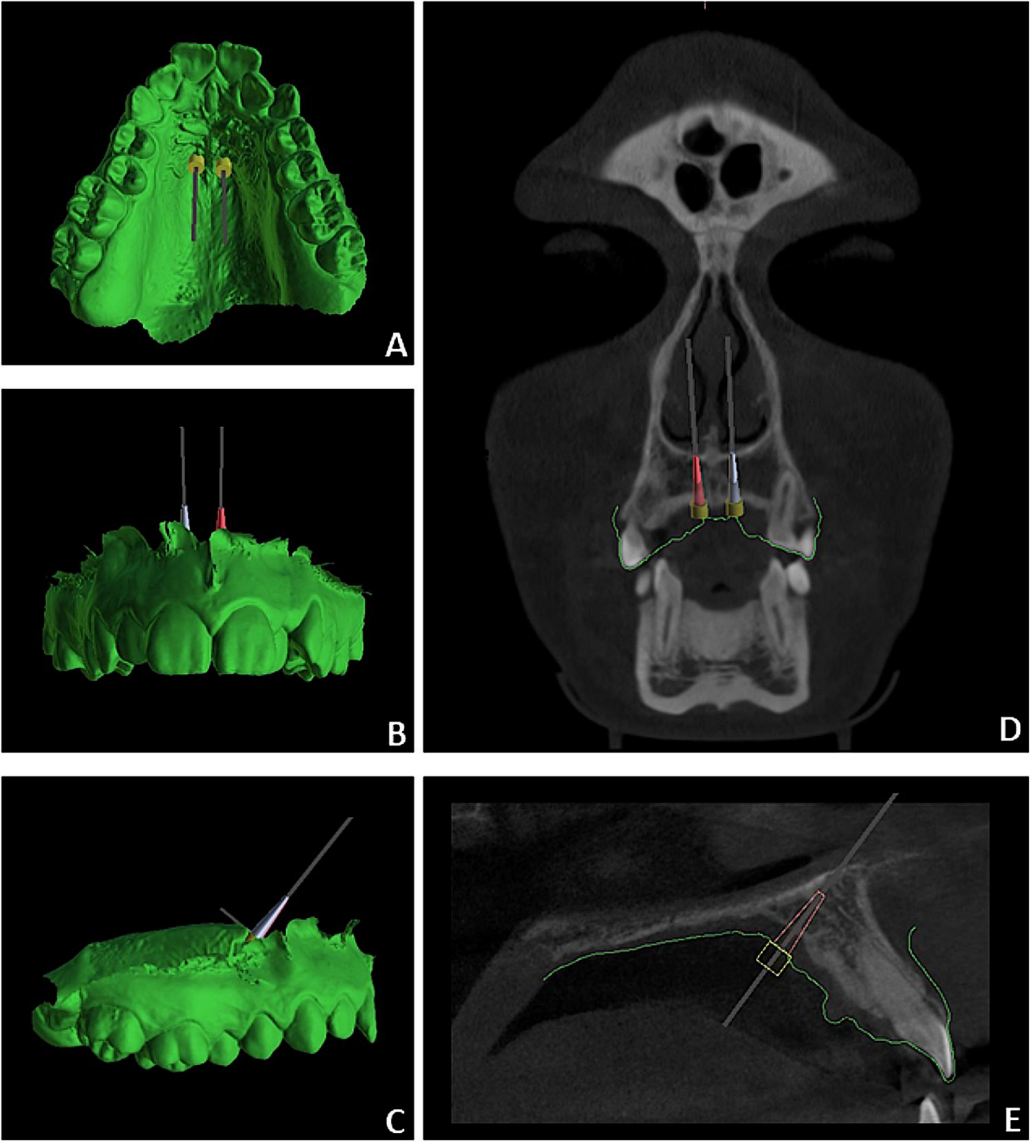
Virtual miniscrew insertion procedure performed using BB-PS (A–C) and CBCT-PS (D,E).
